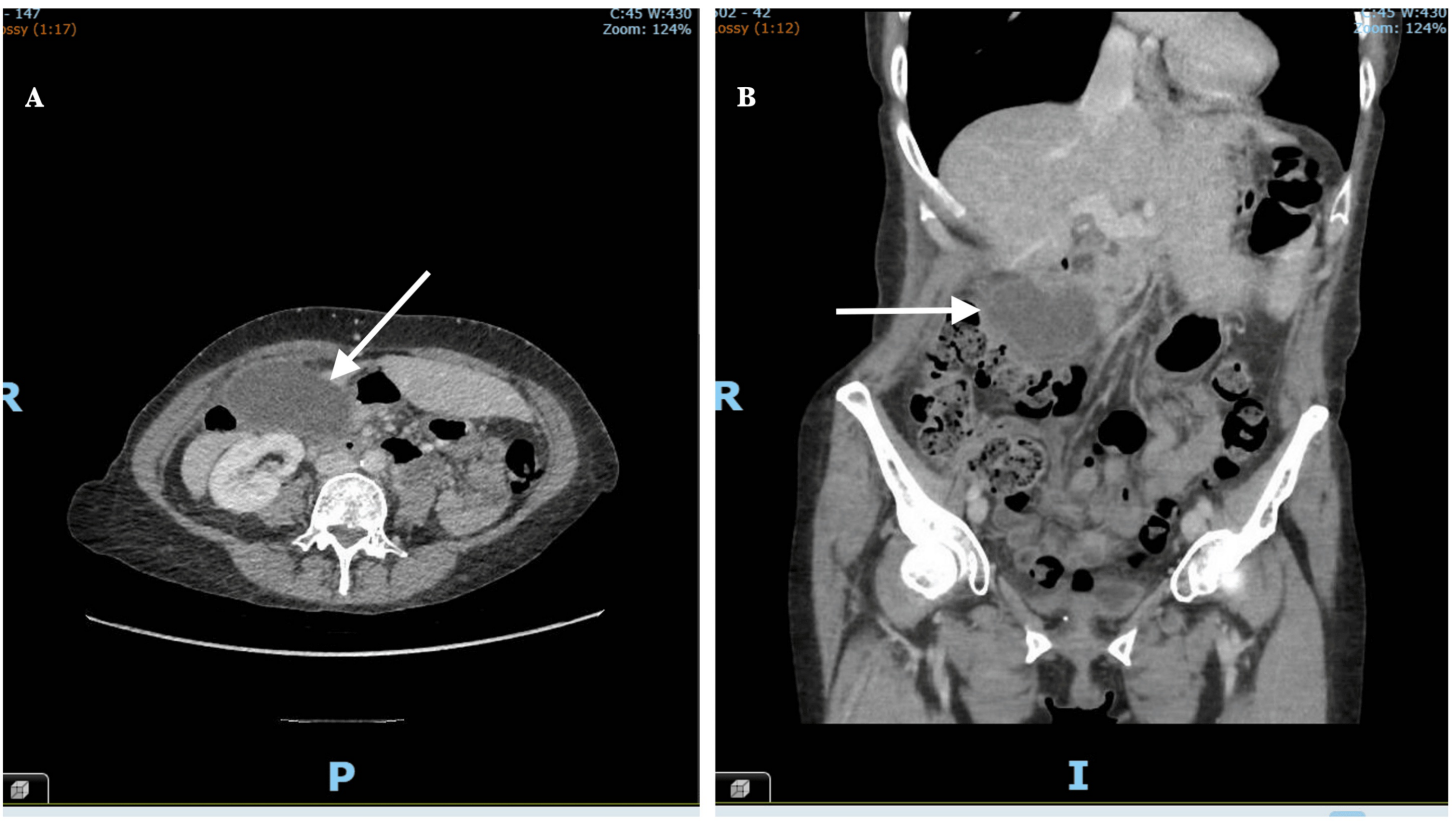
Computed tomography scan showing a thickened gallbladder wall, with no perforation or dilation (A) and (B) Contrast-enhanced computed tomography (CT) images in axial (A) and coronal (B) planes demonstrating a thickened gallbladder wall (arrows) without evidence of perforation or biliary dilation. The surrounding fat planes are preserved, and no gallstones are identified.
